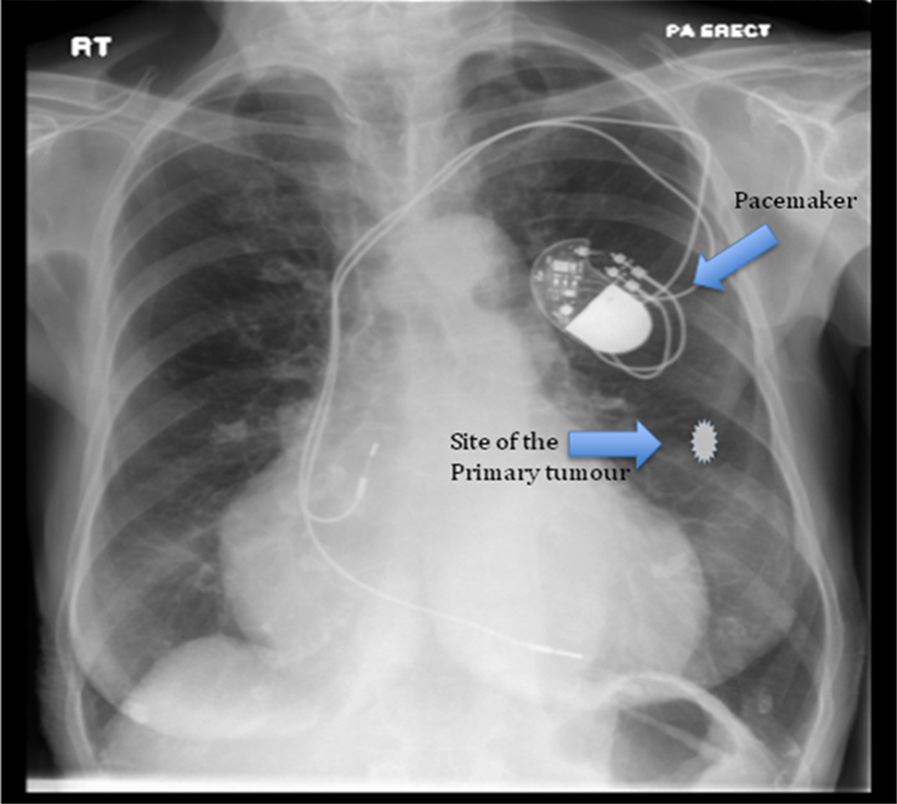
Chest x-ray of the patient showing the pacemaker in situ and the approximate position of the breast cancer.
